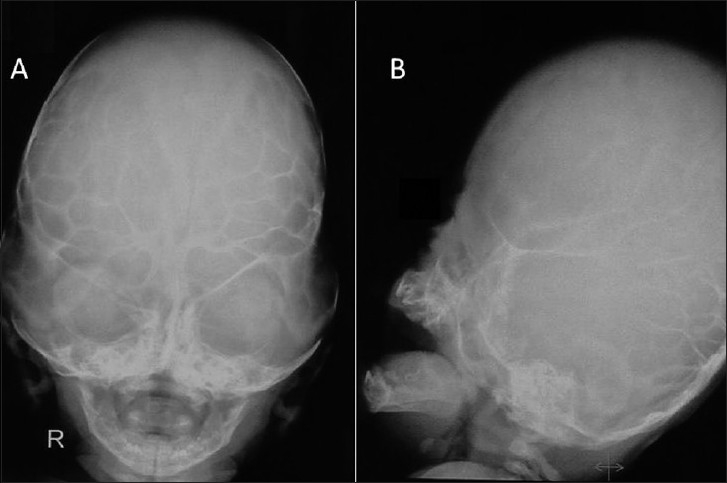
Case 2: (A and B) X-ray (anteroposterior and lateral images) of the skull showed a marked brachycephalic head with midfacial hypoplasia, silver-beaten appearance of the vault and elevation of sphenoid wings.
